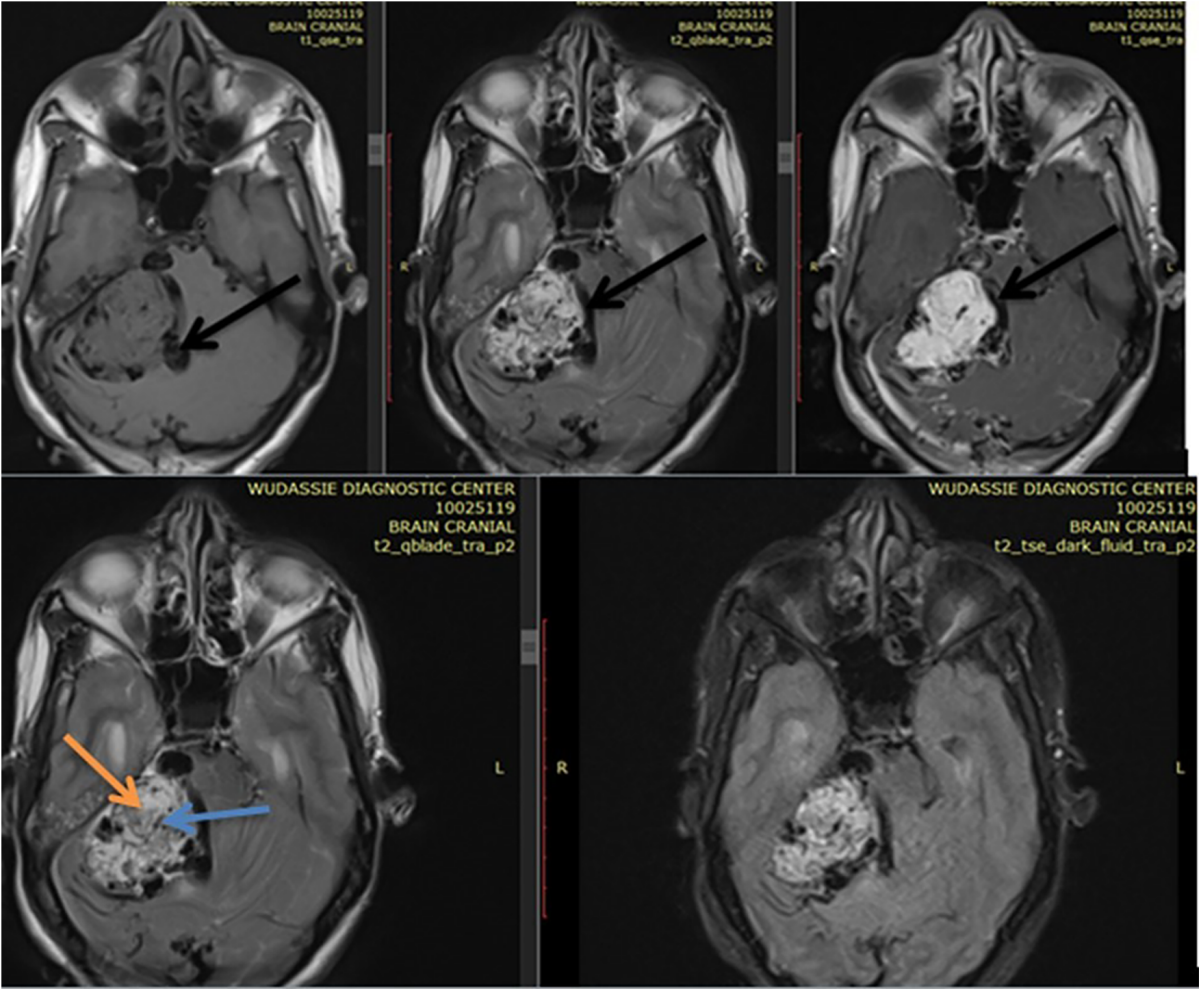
Shows a right side 5 by 5 cm large jugular foramen mass predominantly iso intense on T1 & hyper intense on T2 with extensive multiple & large size intra & peritumoral flow voids as it appears hypointense on both T1 & T2 MRI (see dark arrows of Figure 1). The dark arrow on postcontrast axial MRI in figure shows the “drop out” phenomenon. The tumor also had “salt-and-pepper” pattern (the orange & blue arrow shows the salt & pepper respectively as seen in Figure 1).
